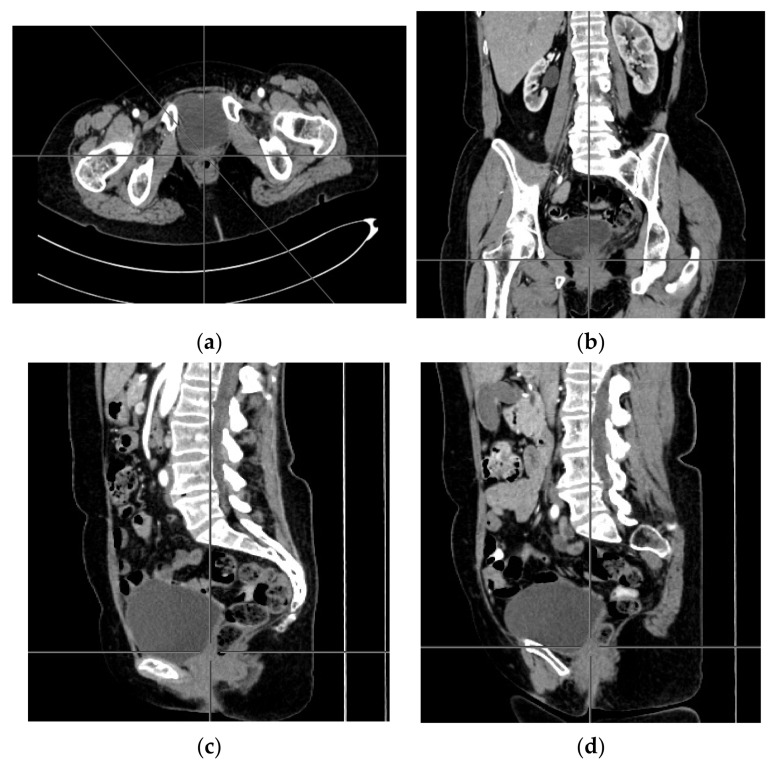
(a–d) CT scans performed before 48 cycle of olaparib treatment.
